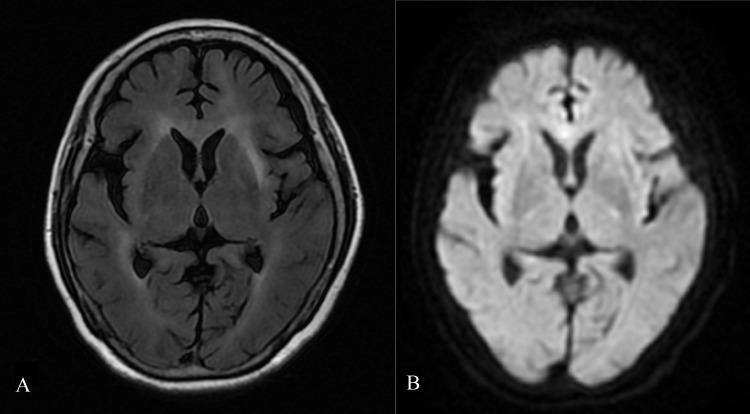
Axial, non-enhanced T2-weighted (A) and DWI (B) MRI image showing brain atrophy and leukoaraiosis. DWI: diffusion-weighted imaging; MRI: magnetic resonance imaging
